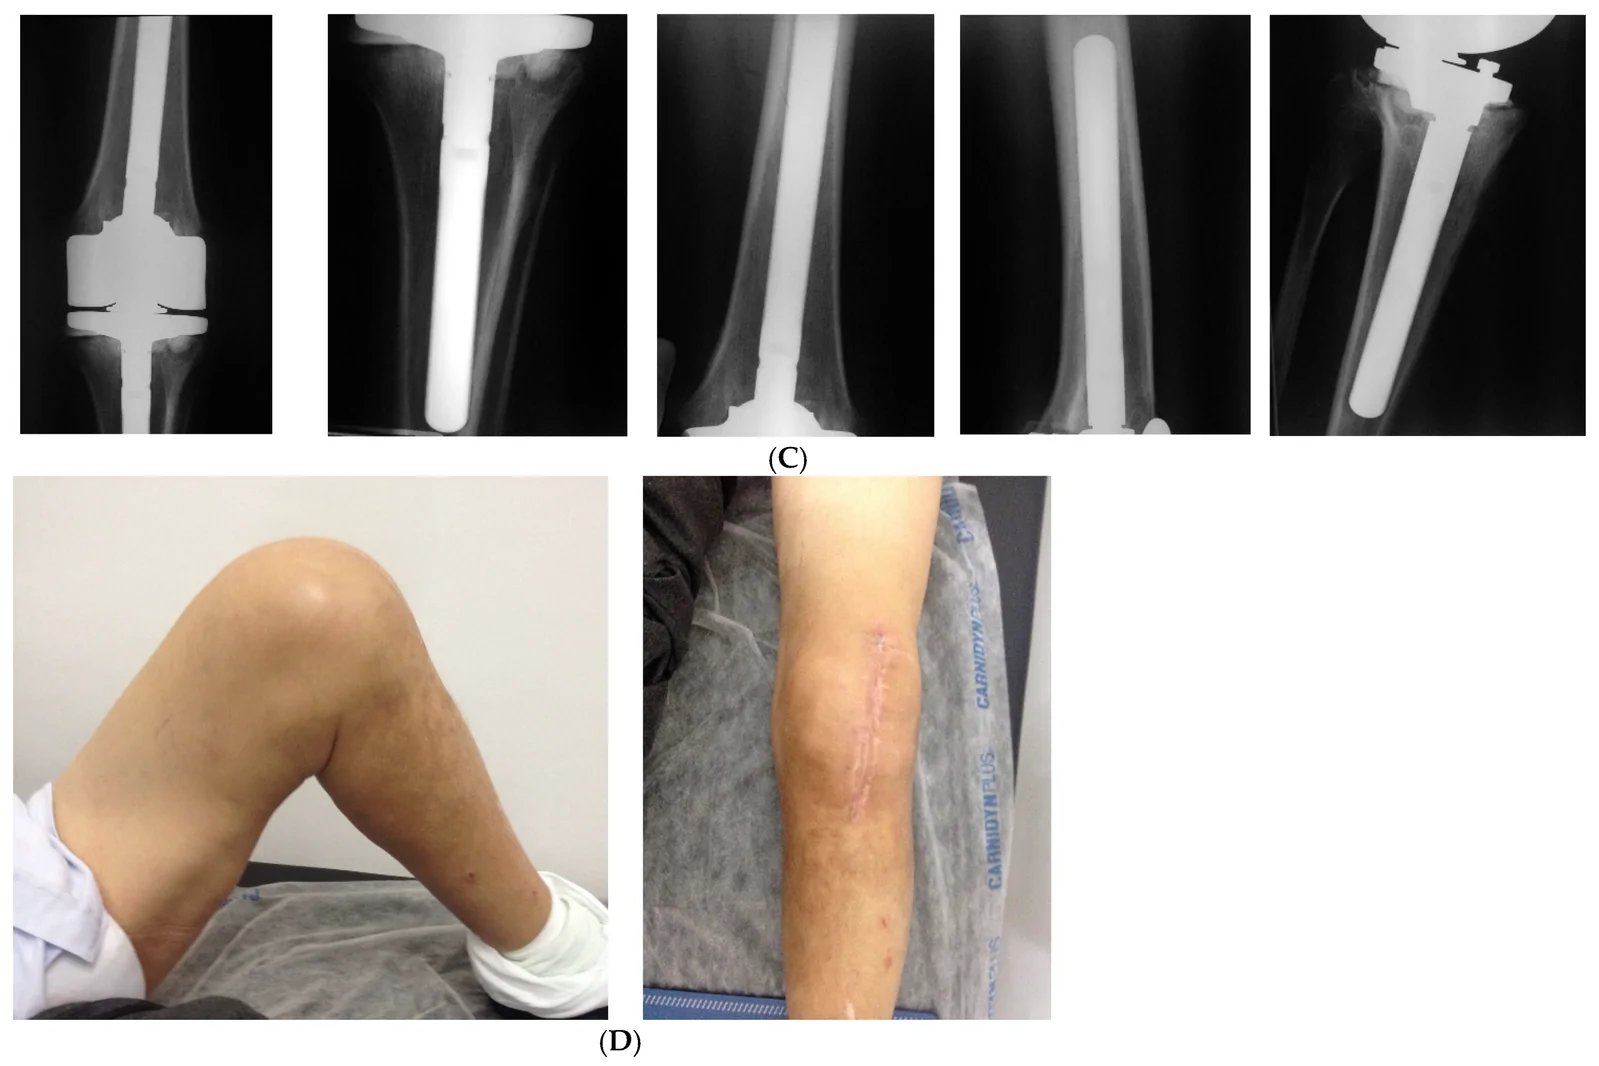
Patient #13. Clinical example of the DAC antibiotic-loaded hydrogel coating application. (A) Pre-operative radiographic examination. Ligament instability. MRSA positive culture. (B) Intra-operative picture, showing DAC hydrogel application on the femoral implant, prior to its insertion. (C) Radiographic examination 6 years after surgery. (D) Clinical outcome.
